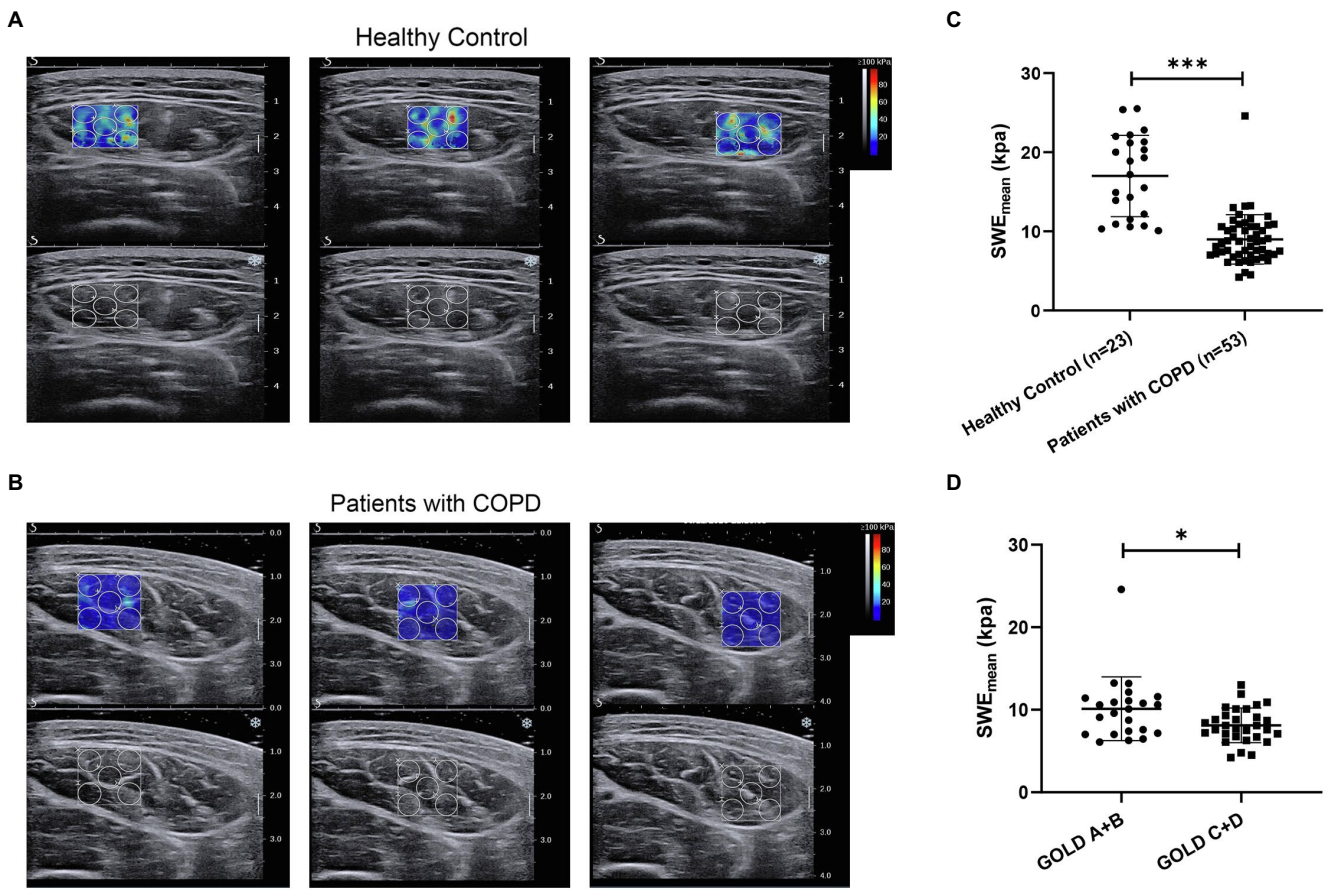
The difference in the mean elasticity indices of rectus femoris ultrasound elastography in patients with chronic obstructive pulmonary disease (COPD) and healthy controls. (A) Representative SWE images of the rectus femoris and grayscale ultrasound images in healthy controls; (B) Representative SWE images of rectus femoris and grayscale ultrasound images in patients with COPD; (C) Difference in mean elasticity index (SWE_mean_) in patients with COPD and healthy controls; and (D) Difference in SWE_mean_ in patients with global initiative for chronic obstructive lung disease (GOLD) A and GOLD B and in patients with GOLD C and GOLD D. SWE_mean_: mean SWE elasticity index. p*<0.05; p*<0.001.
